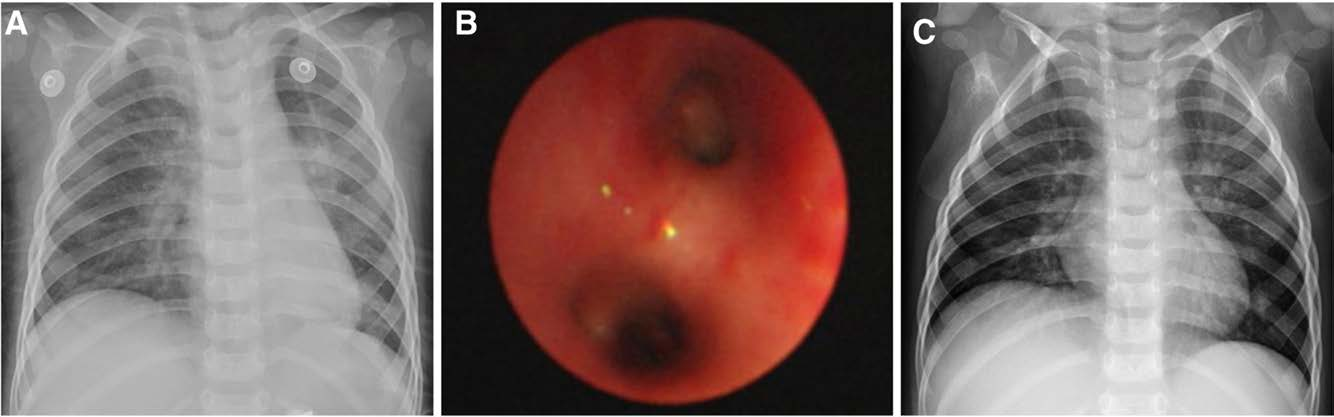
(A) Subsequent electronic bronchoscopy revealing no casts in the bronchus; (B) chest radiograph post-bronchoscopy indicating a significant improvement in atelectasis in the left lung; (C) chest radiograph displaying normal results 2 weeks after discharge.
